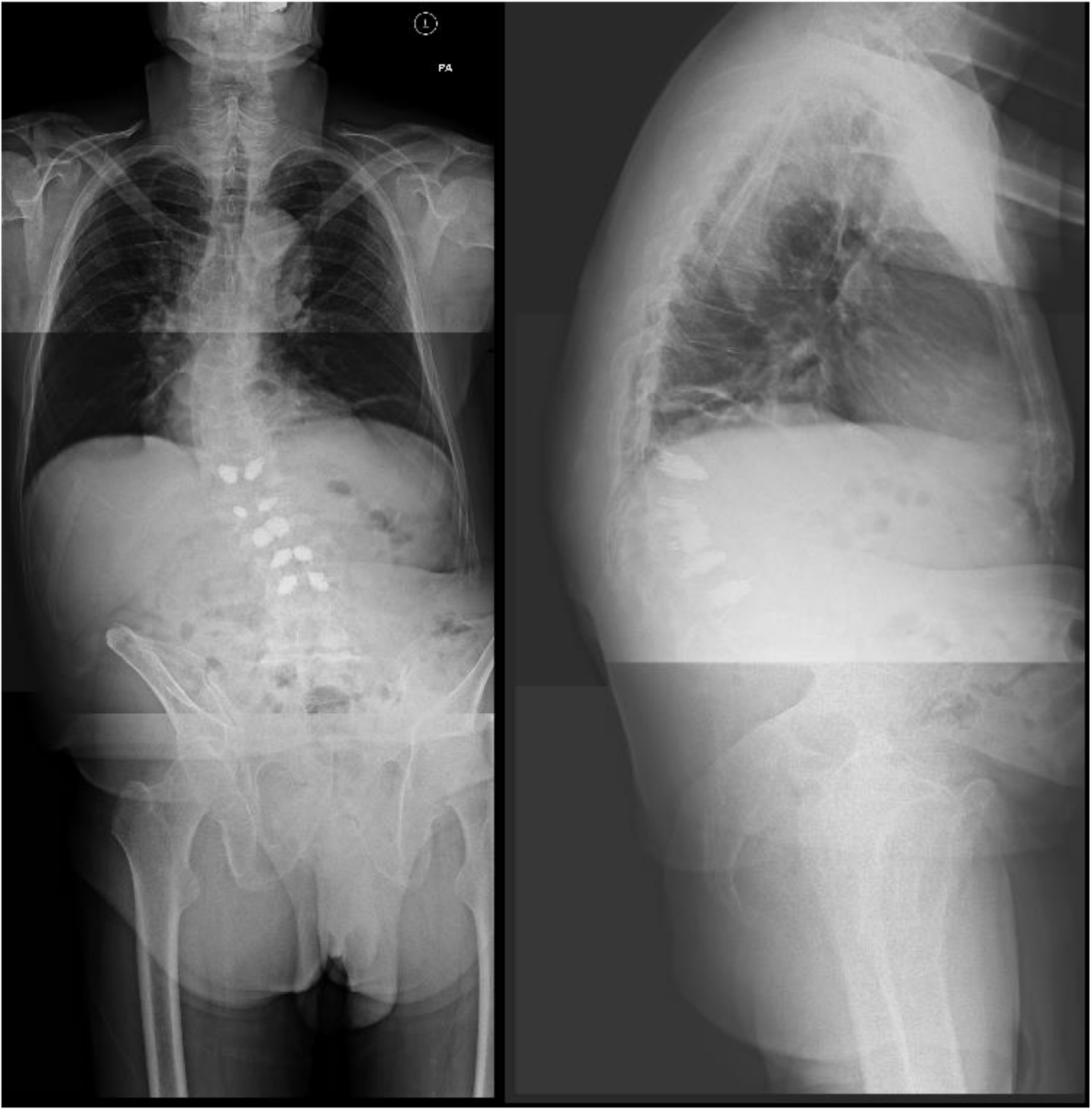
Six week postoperative PA and lateral whole spine radiographs showing multi-level instrumentation with the Osseofix™ implant.
